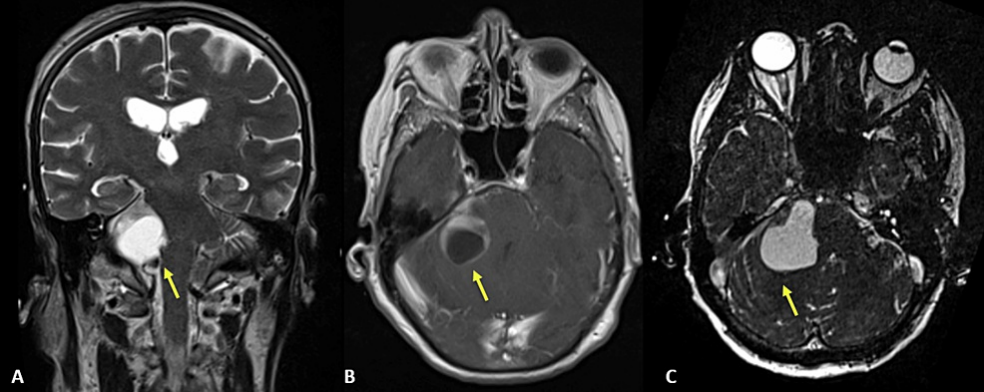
Cranial MRI showing apparently cystic cerebellopontine angle lesion, causing brainstem compression and IV ventricle collapse. (A) Coronal T2; (B) gadolinium-enhanced axial T1; (C) axial CISS sequence. Yellow arrow indicating the lesion.
